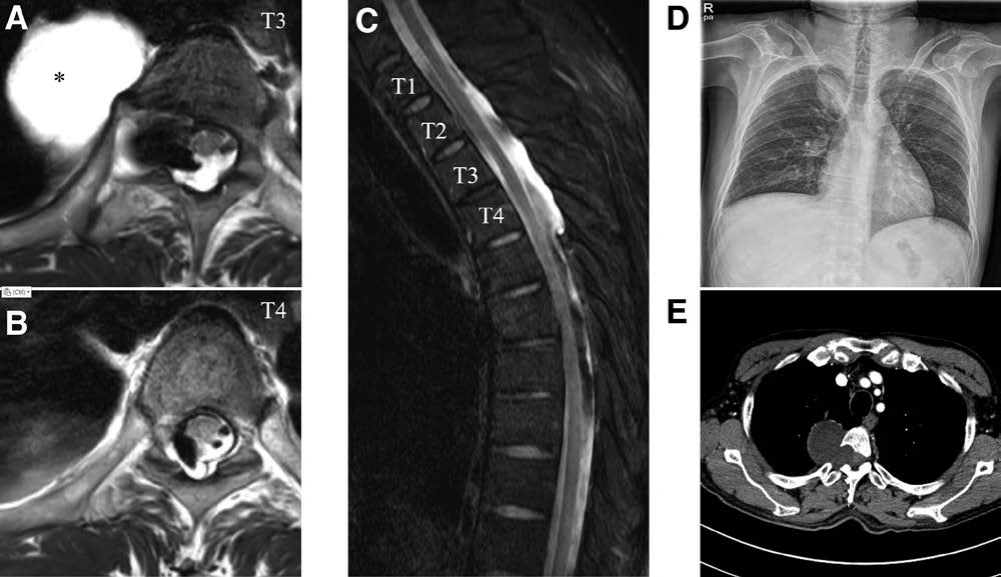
Initial radiologic findings of the patient. Axial and sagittal T2-weighted MR images show a 4.5 × 5.0 × 4.8 cm sized mass with high signal intensity (black asterisk) in the right paravertebral area at the T3-T4 vertebral level, and the normal-appearing spinal cord (A–C). Chest X-ray shows an oval high-density lesion in the upper right lung field (D). Chest computed tomography shows a dumbbell-shaped low-density mass lesion with the bony remodeling of the T3-T4 vertebrae and widened intervertebral foramen (E). MR = magnetic resonance.
